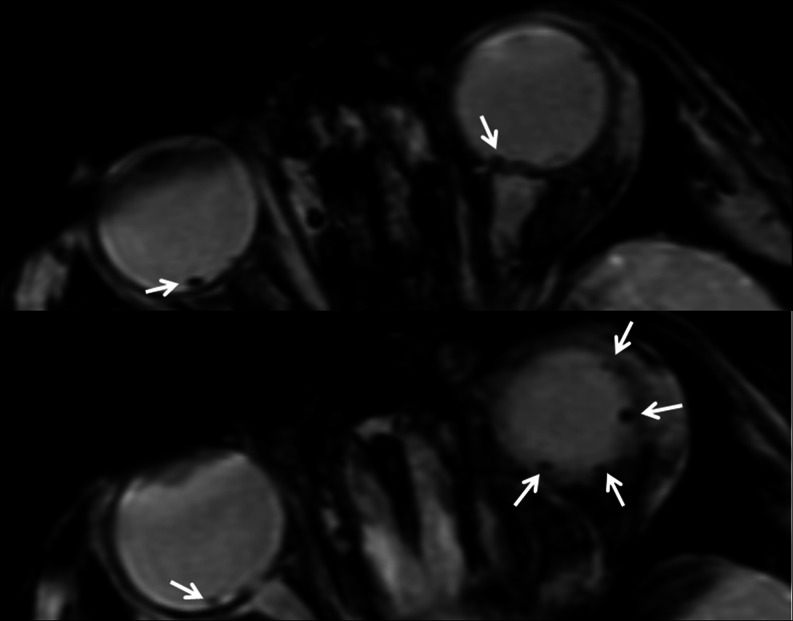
MRI on patient 1. Multiple areas of low signal intensity consistent with retinal hemorrhages are noted on SWI images (*arrows*) on a 3T magnet on the standard brain MRI protocol. Intraretinal hemorrhages were identified in the right fundus, and intraretinal and preretinal hemorrhages were identified in the left fundus on DFE (not shown)
